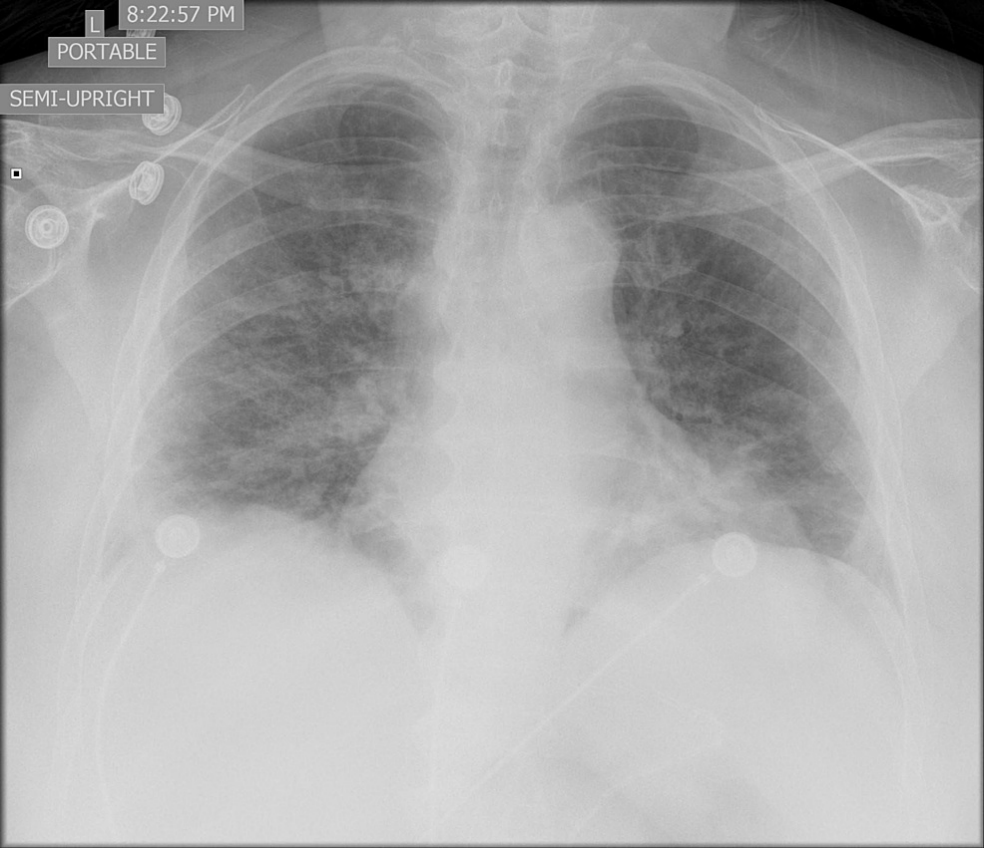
Chest x-ray which showed bilateral diffuse consolidation consistent with multifocal pneumonia
